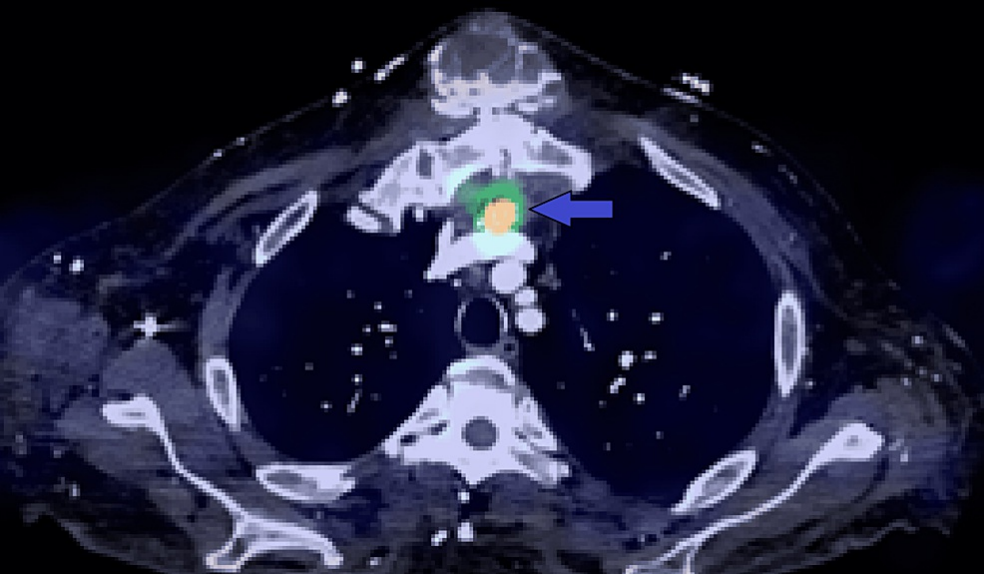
Retrospective image fusion of low VMI from SDCT and SPECT data shows the anterior mediastinal nodule and radiotracer uptake more clearly (blue arrow).
